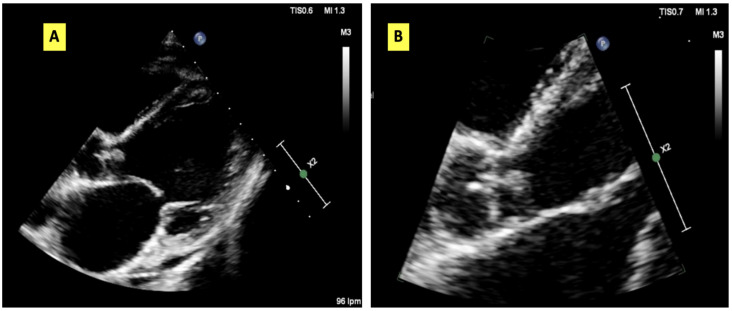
Aortic vegetation visualised using transthoracic echocardiography. (A) 3-chamber apical plane. (B) Enlarged image of the vegetation.
